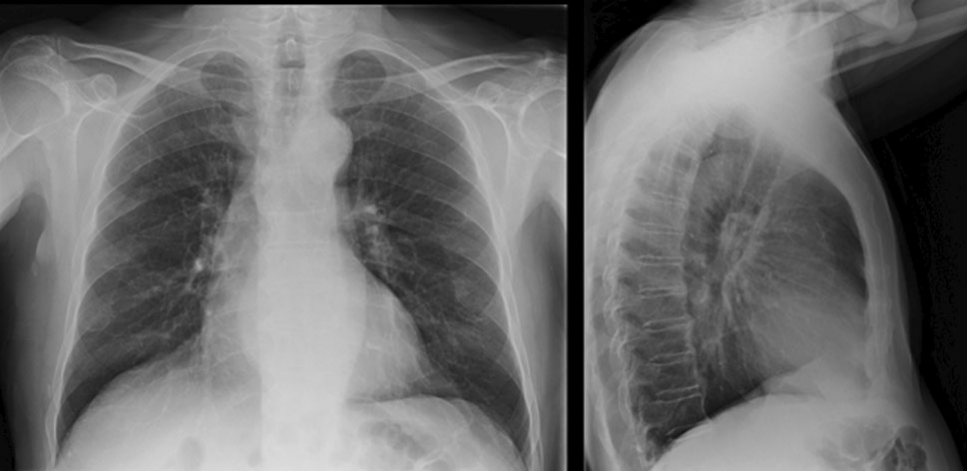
Plain chest X-ray showing a convex opacity overlapping the right mediastinal contour. The gastric bubble is also absent
